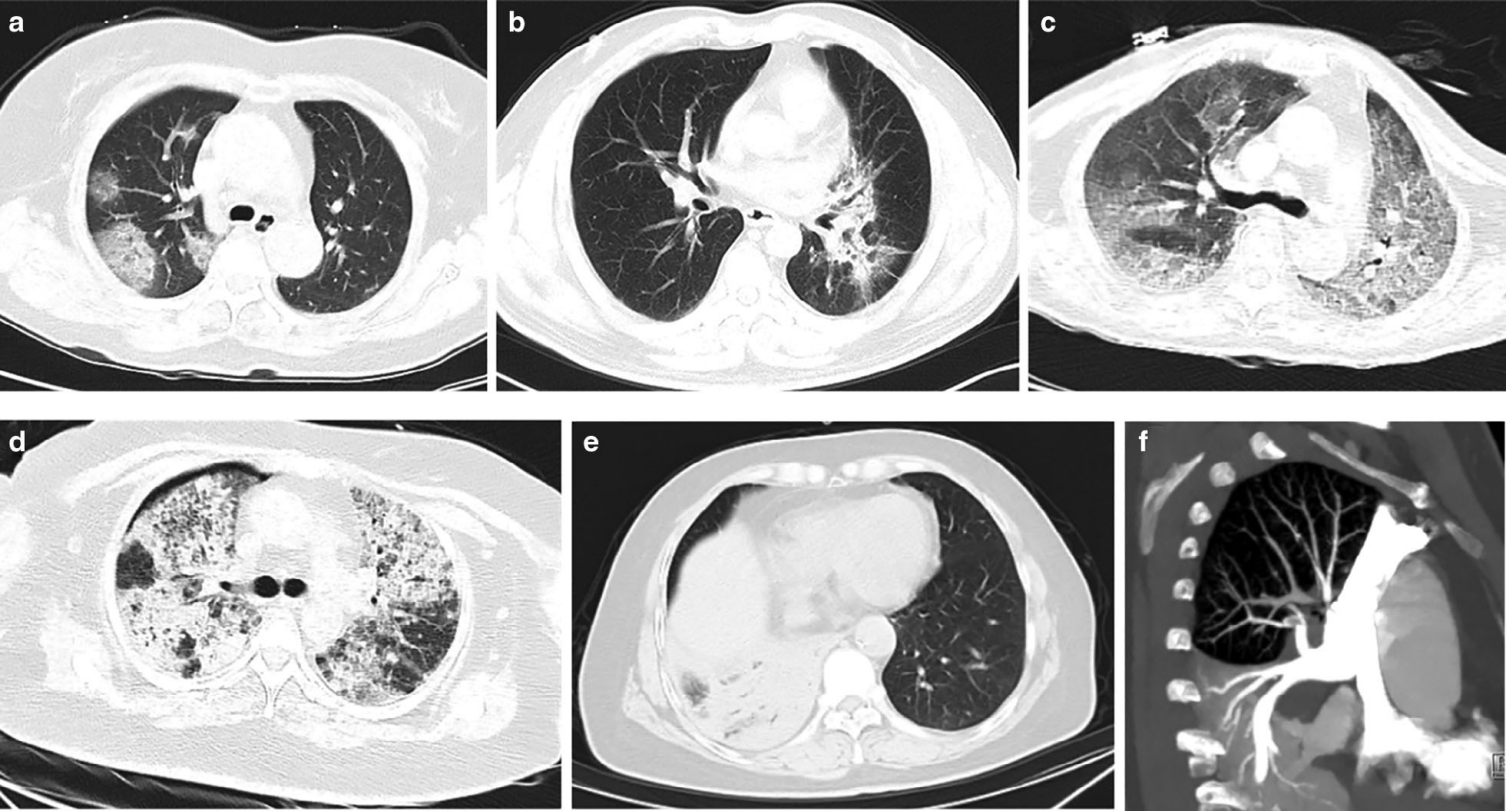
Chest computed tomography (CT )images of COVID‐19 and lung cancer patients. (a) Transverse chest CT image from COVID‐19 patient showing ground‐glass opacity (GGO). (b) Transverse chest CT image from lung cancer patient with radiation pneumonitis showing GGO consistent with the radiation field. (c) Transverse chest CT image from lung cancer patient with ICI‐P showing diffuse GGO. (d) Transverse chest CT image from pneumonic‐type lung carcinoma with tumor progression showing diffuse GGO with multiple bubble‐like low attenuation. (e) Transverse chest CT image from lung cancer patient with dyspnea showing right lower pulmonary obstructive pneumonia and filling defect of the right superior pulmonary artery on CTPA (f) indicating pulmonary embolism.
